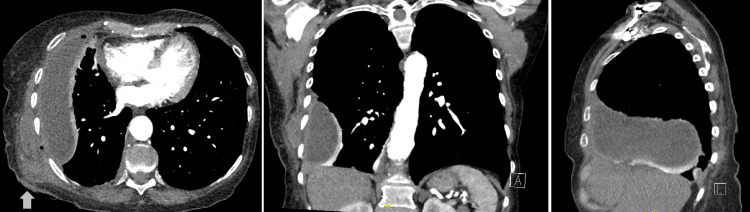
CT imaging on re-admission. Note the extension of fistulous tract to the subcutaneous tissue and skin
